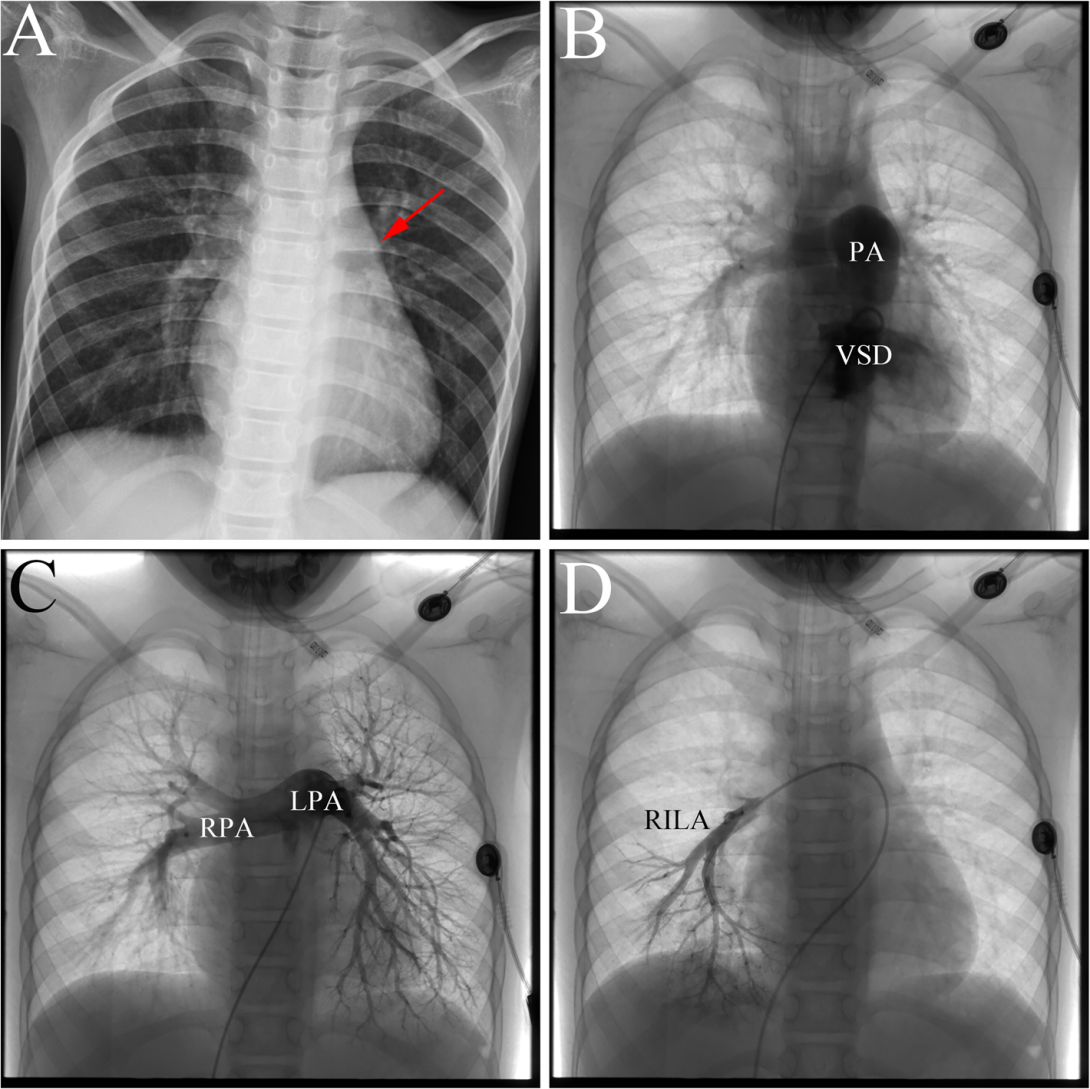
The representative image of PAH-VSD. a: Chest X-ray confirmed a bulging pulmonary artery at the upper left cardiac border; b: Right heart catheterization revealed a lager VSD; c and d: Pulmonary arteriography showed that the degree of pulmonary capillary filling decreased. PAH: pulmonary arterial hypertension; VSD: ventricular septal defect; PA: pulmonary artery; RPA: right pulmonary artery; LPA: left pulmonary artery; RILA: right inferior lobe artery
